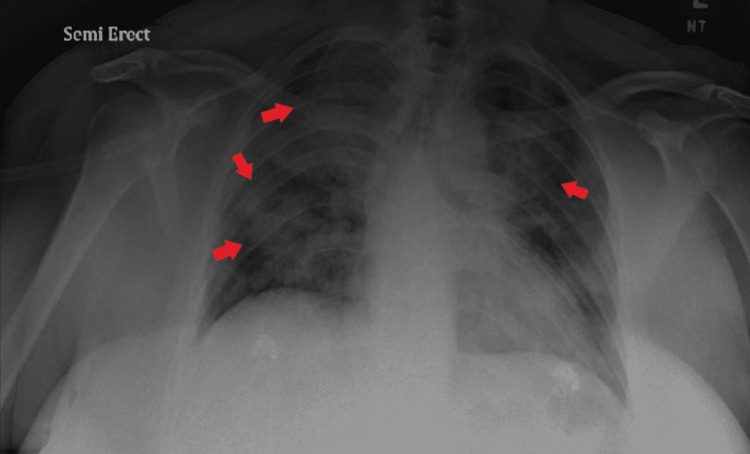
Chest X-ray revealing patchy ground-glass opacities within mid and lower lung fields.
